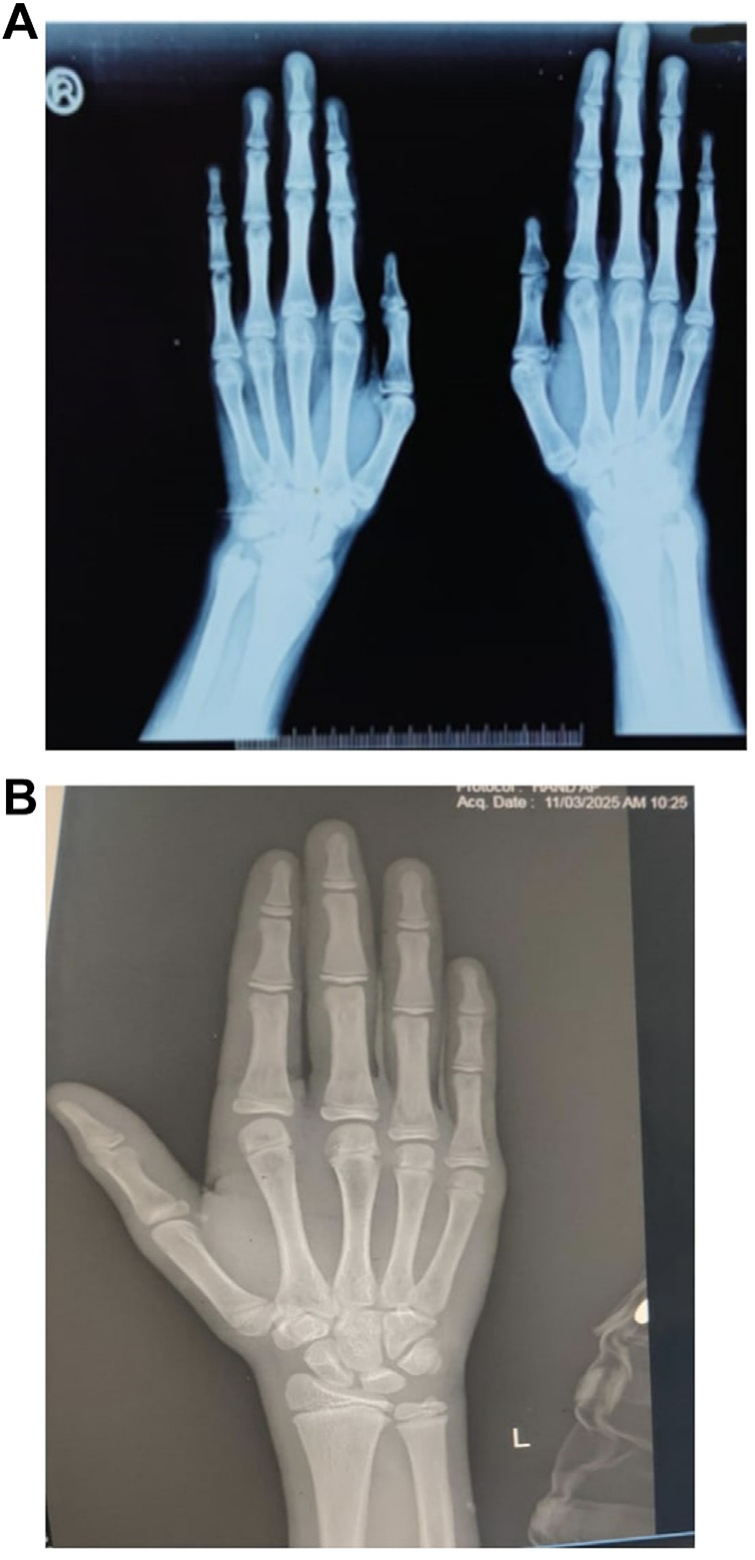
A*: x-ray of the hand showing ARACHNODACTYLY, *B*: Normal x-ray of the hand of a 17-y-old male.
